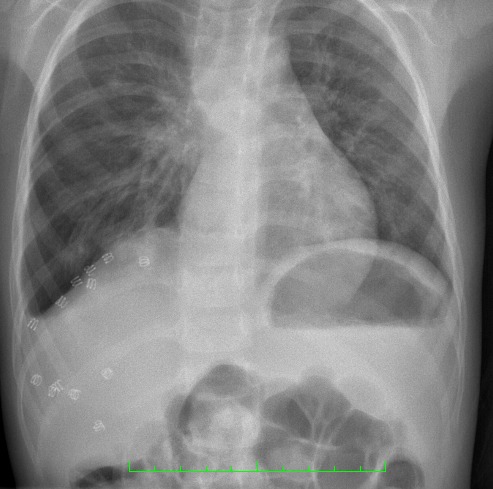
Figure 3: Chest X-ray. Three-year follow-up
